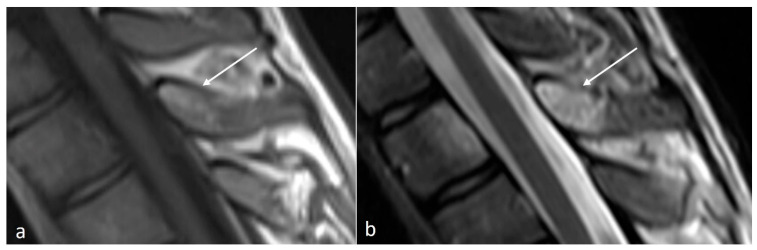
Sagittal T1 (a) and T2 (b) MRI images demonstrating a thoracic spinous process haemangioma (white arrows). Note the presence of high T1 and T2 signals, which are characteristic of a typical haemangioma due to the fat content.
